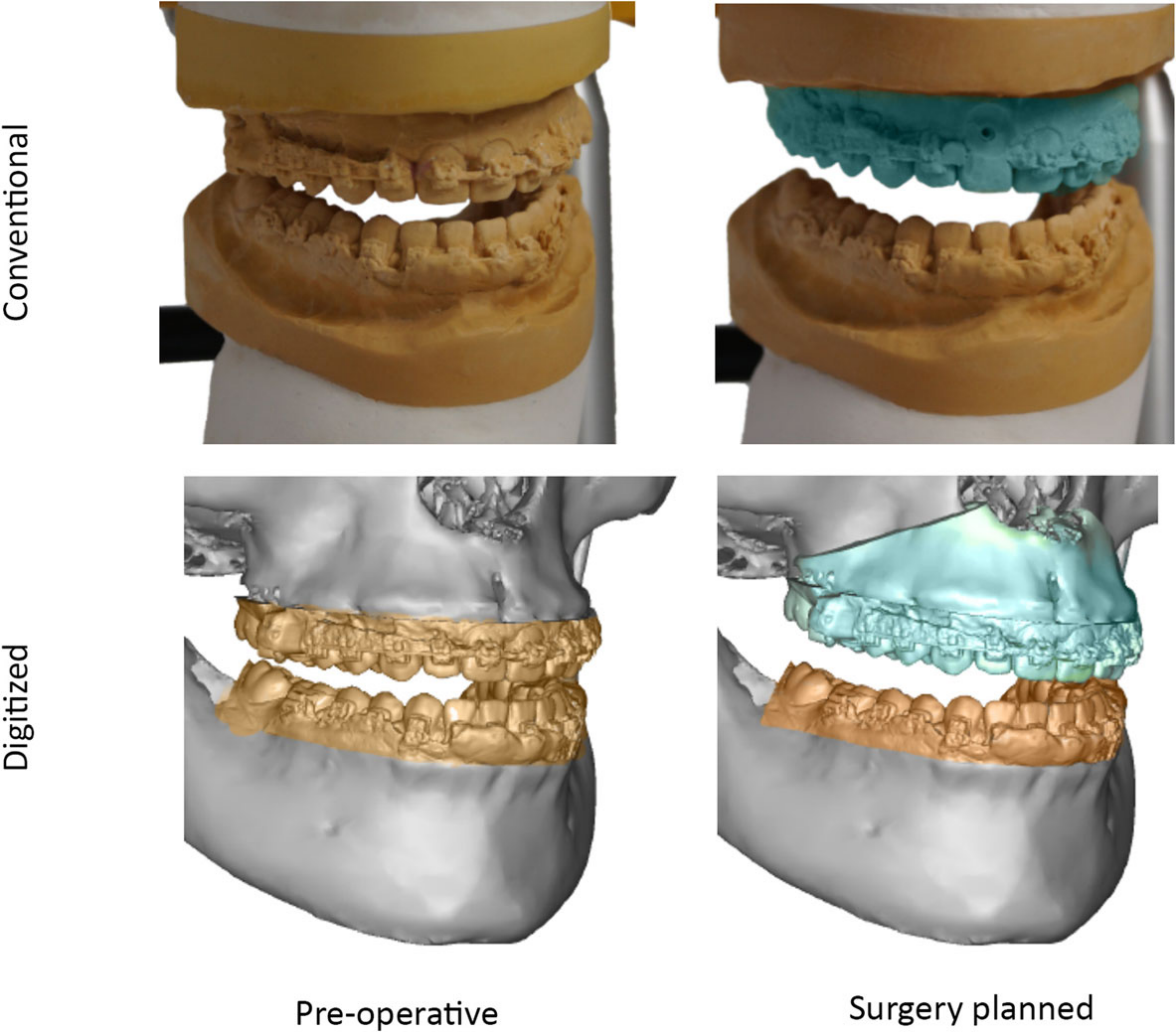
Digital reconstruction of the conventional surgery planning: Left side: Alignment of the preoperative CBCT scan and the dental casts with the upper and lower jaw in the preoperative, centric position. Right side: Reconstruction of the planned postoperative maxillary position by alignment of the preoperative mandible and the osteotomized maxilla as bony structures and the upper jaw in the postoperative and lower jaw in the preoperative position as dental casts. The mandible served as fixed reference while the maxilla moved in the planned postoperative position
